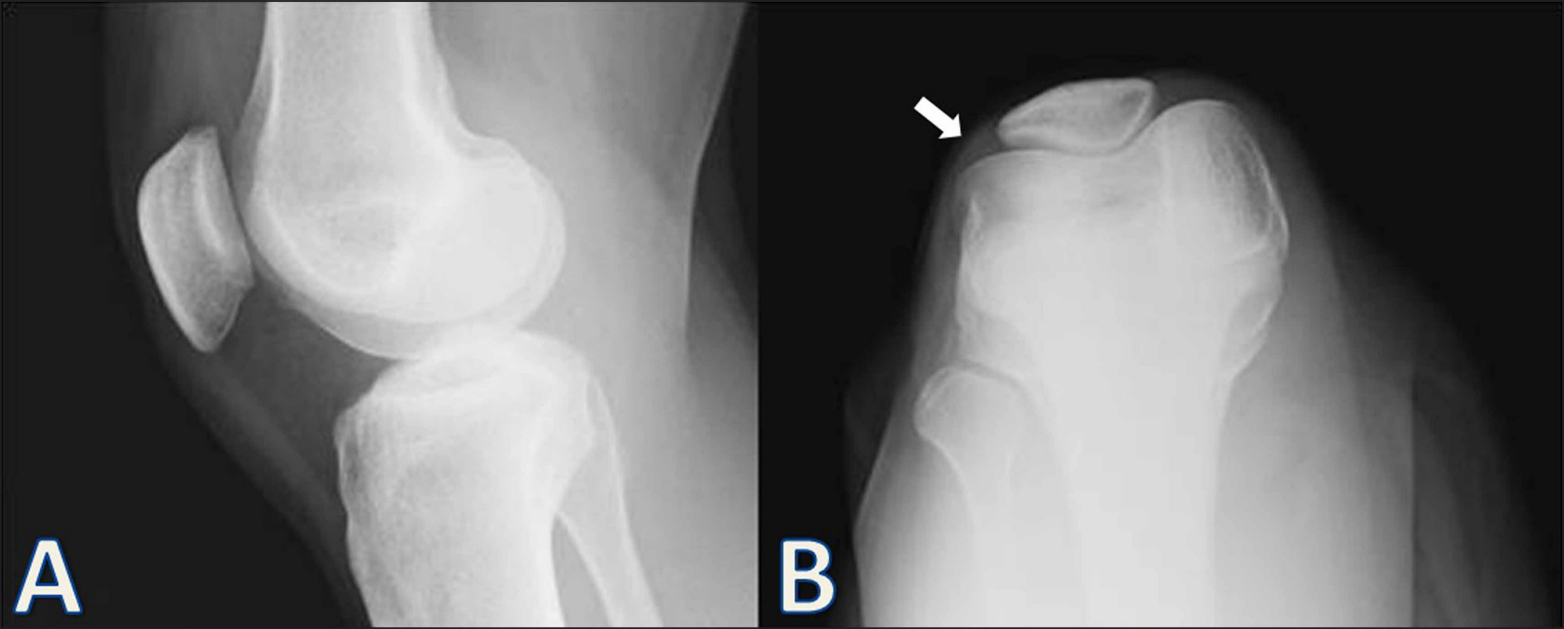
Lateral (A) and skyline (B) plain orthogonal radiographs of the right knee demostrate no bone abnormalities. The soft tissue mass (white arrow) is best seen on the skyline radiographic view.
